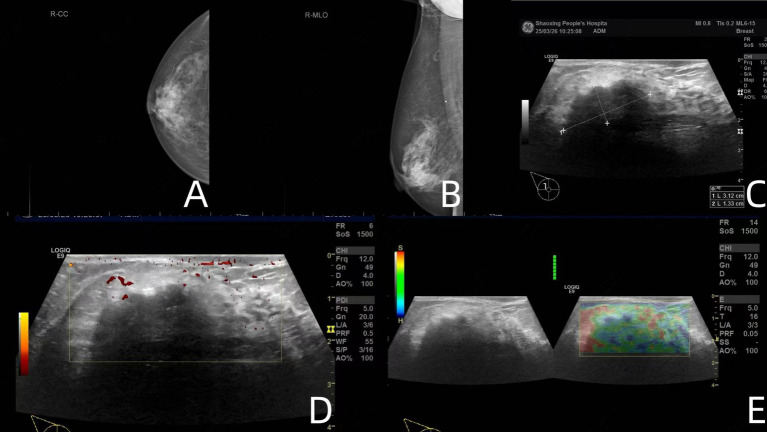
Mammography demonstrated a nodular hyperdense lesion measuring approximately 28 × 23 mm behind the right nipple, characterized by partially ill-defined borders and punctate calcification (A,B). Breast ultrasonography further revealed a 31 × 13 × 29 mm hypoechoic nodule with irregular shape and peripheral blood flow signals (C–E).
